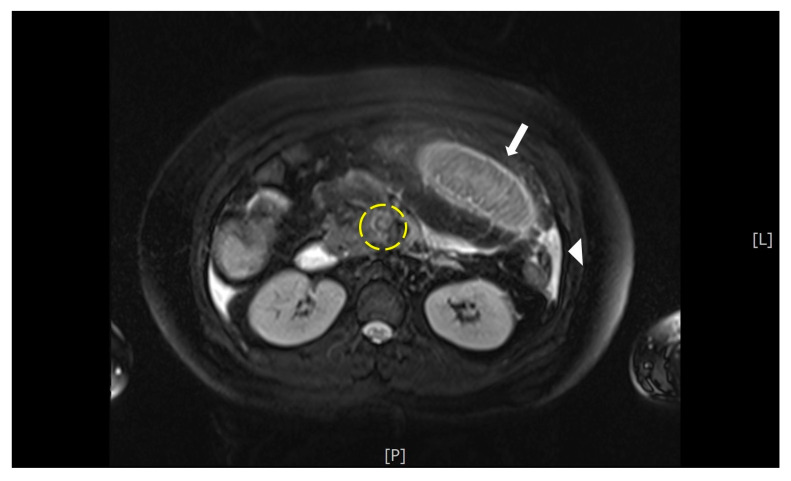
MRI This T2- fat saturation image demonstrates a hyperintense filling defect within the superior mesenteric vein (yellow circle). There is also thickening of the wall of a small bowel loop (arrow), and a small amount of ascites (arrow head).
